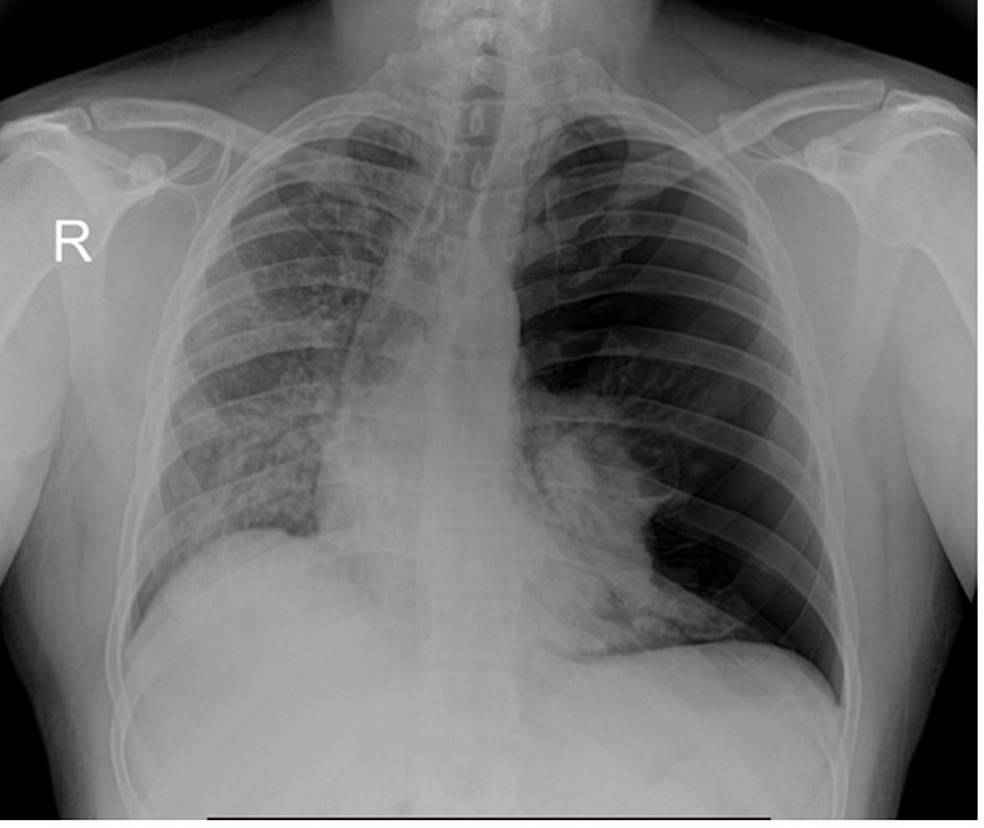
Portable chest X-ray of a large left-sided pneumothorax with mediastinal shift which required the placement of a thoracostomy tube.
